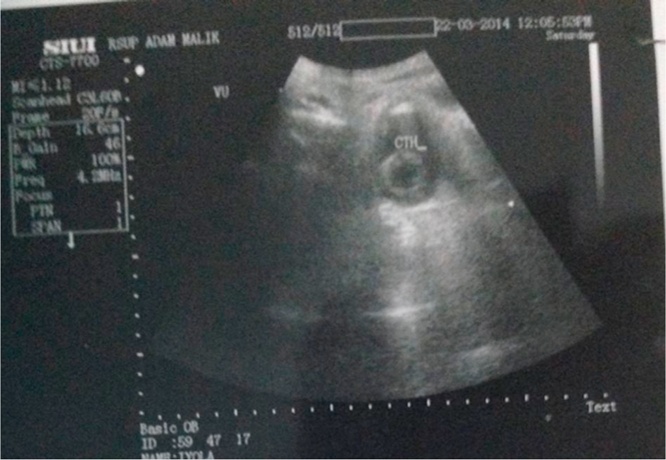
The patient’s ultrasound before bladder augmentation.
